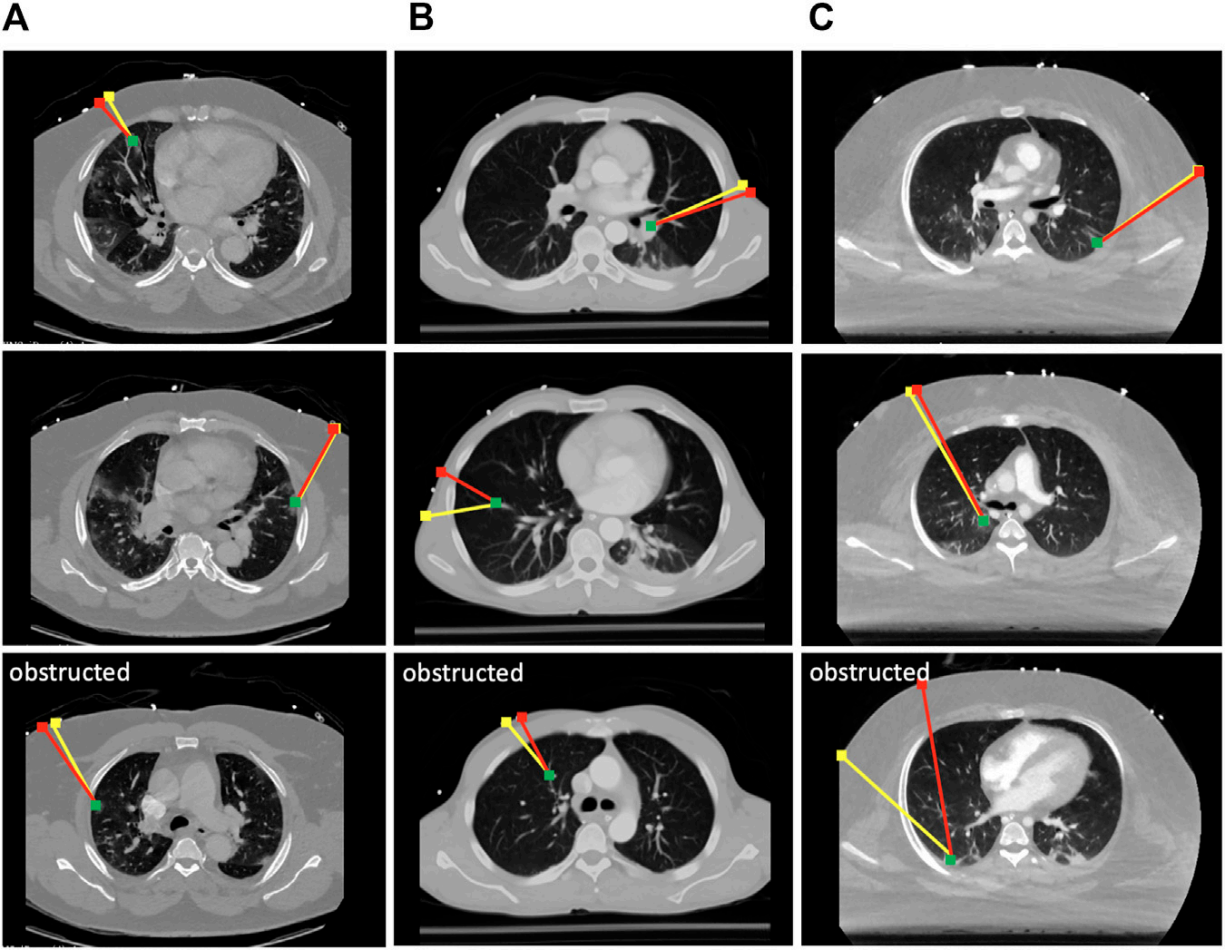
Selected results from the optimal scanning algorithm compared to medical expert’s selection. The yellow lines mark the expert’s proposed paths, and the red lines are the ones proposed by the algorithm. The green dots are the target points that need to be imaged in the lungs. Paths returned by the expert that were obstructed by the ribs are marked as such. Each column of results corresponds to a CT scan of a different patient. (A) Results from COVID-19 patient 1; (B) results from a healthy patient; (C) results from COVID-19 patient 2.
